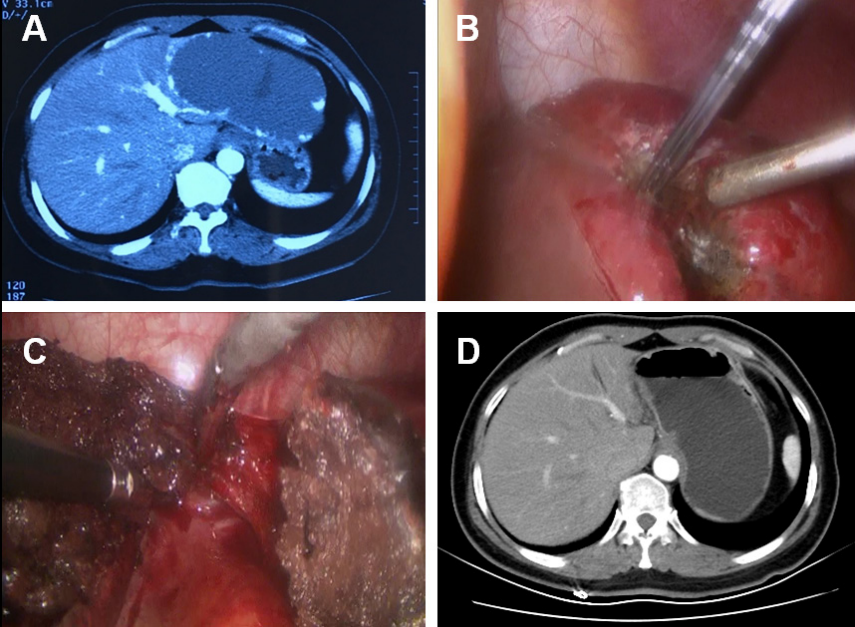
CT and intraoperative photos of case 1 A. Contrast-enhanced CT demonstrated a 12.0-cm hepatic hemangioma located in the lateral left lobe of liver. B. Intraoperative photo showed the hemangioma at the resection margin shrank significantly after radiofrequency ablation. C. The hemangioma was removed and a 1.0-cm band of ablation-coagulated hemangioma was left in place. D. Contrast-enhanced CT showed that no residual tissue of the hemangioma exist in the tumor-dissected area.
